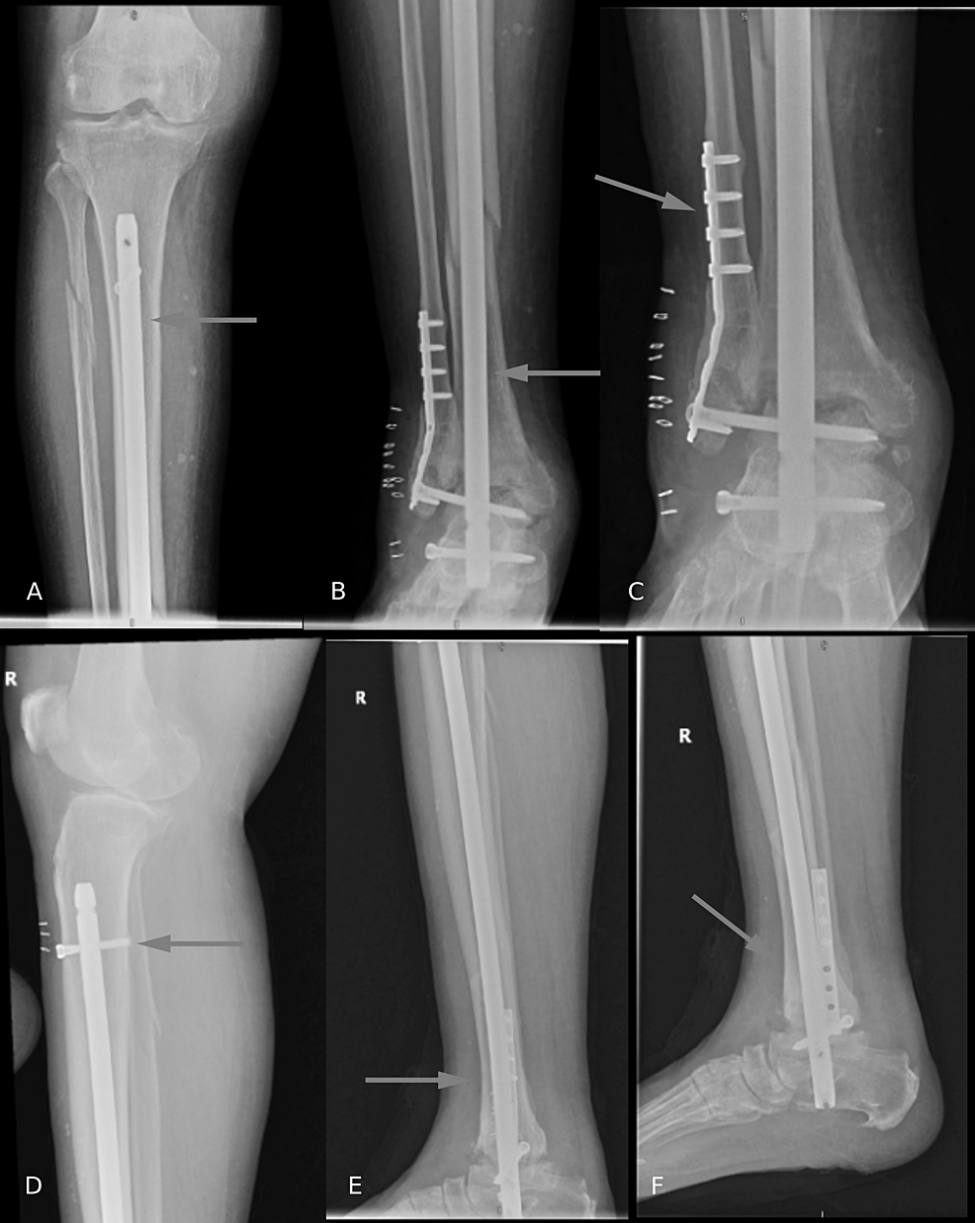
X-rays after the surgery. (A, B) Anteroposterior view of the right tibia and fibula, (C) anteroposterior X-ray of the ankle joint, (D, E) lateral views of the leg, (F) lateral view of the ankle
